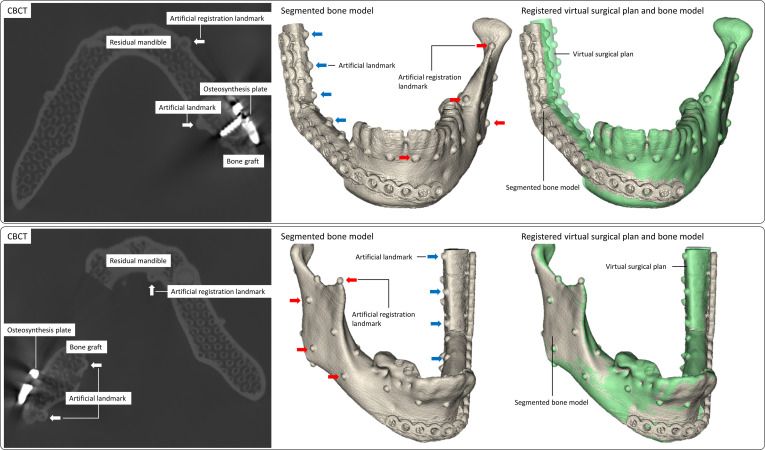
Postoperative imaging. Two study cases reconstructed with 2 fibular segments on the right side of the mandible (top) and the left side of the mandible (bottom). Left: Postoperative cone beam computed tomography (CBCT) scans of the study cases. Middle: Segmented and 3-dimensional (3D) triangulated bone models (gray), with artificial landmarks for measuring accuracy (blue) and artificial registration landmarks (red) of the study cases. Right: Registered virtual surgical plan (green) and bone model (gray) of the study cases.
